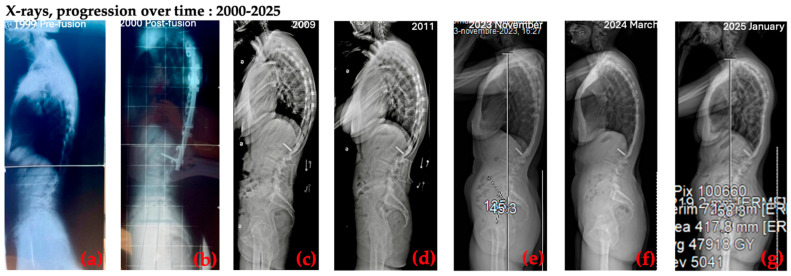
Sagittal X-ray progression over time: (a) pre-surgery, 1999; (b) post-surgery, 2000; (c,d) sagittal postural deterioration, 2009 and 2011; (e) sagittal postural improvement, after 1 year Schroth + brace; (f) sagittal postural improvement, after 17 months Schroth + brace; (g) sagittal postural improvement, after 28 months Schroth + brace.
